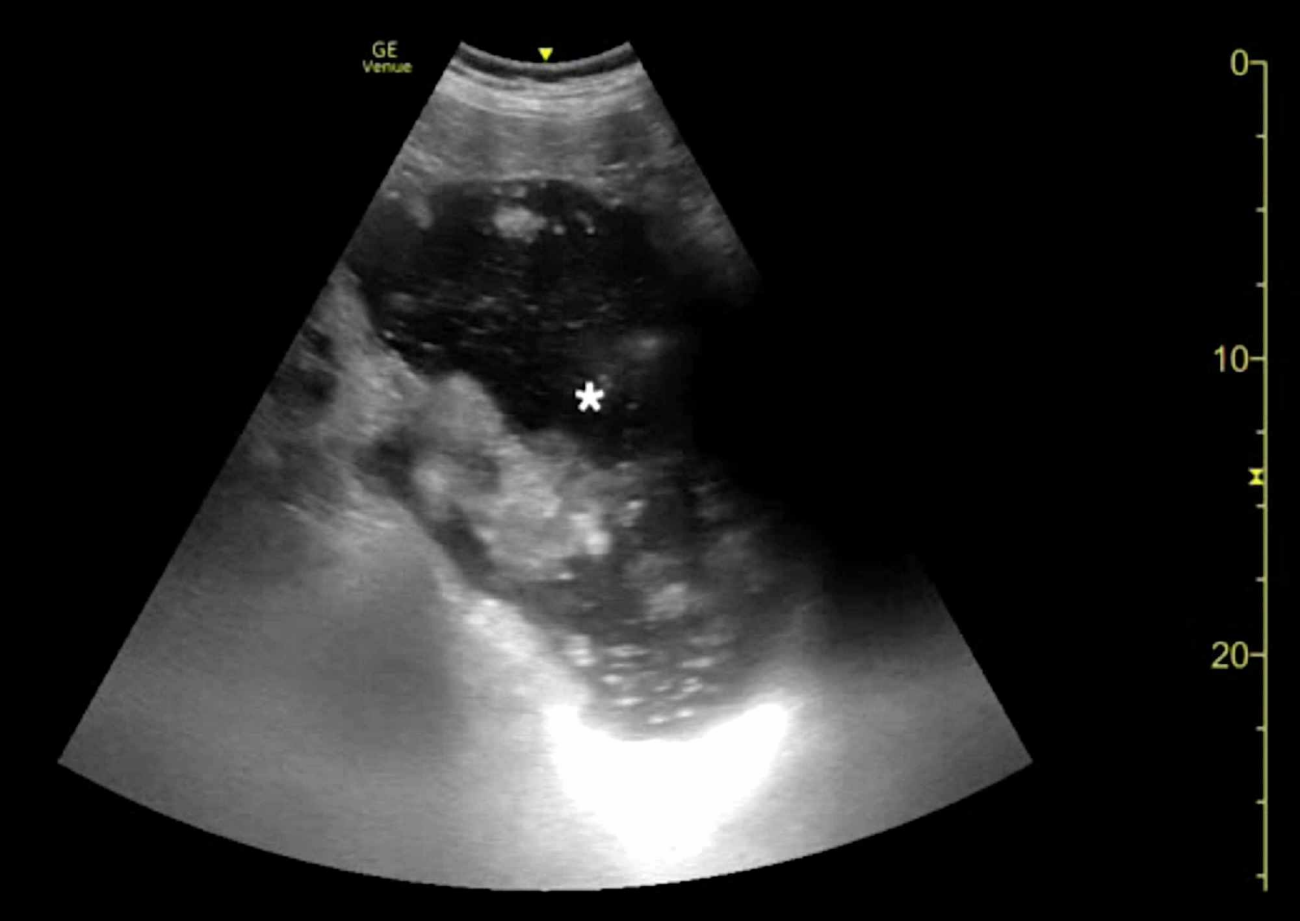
Point-of-care ultrasound of gastric volvulus demonstrating a distended, fluid-filled stomach (asterisk).
